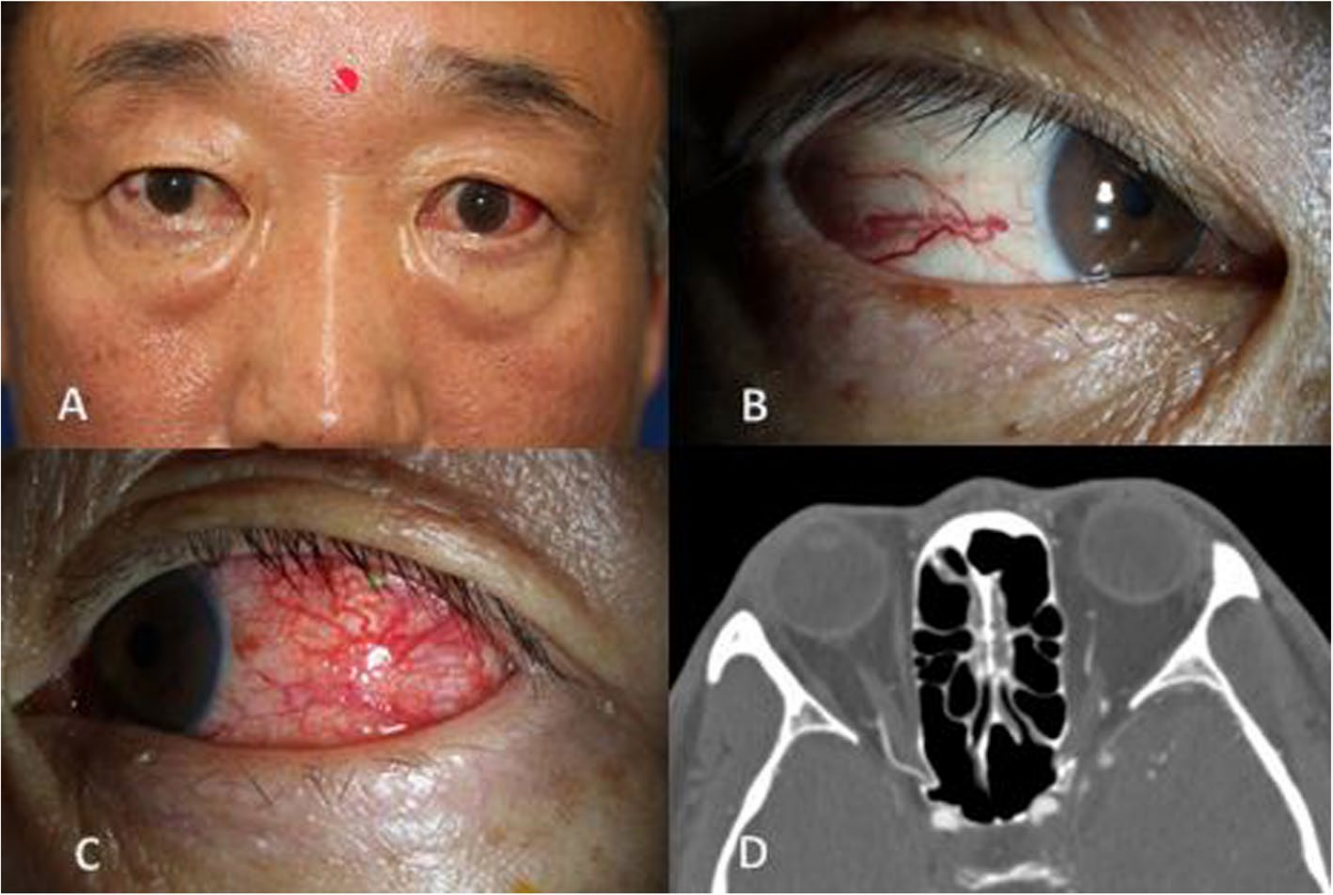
Photographs and CTA image of the case 3 patient. A The patient presented with conjunctival injection of his left eye. B, C Engorged corkscrew conjunctival vessels in both eyes. D CT angiography reveals no enlargement of the SOV
